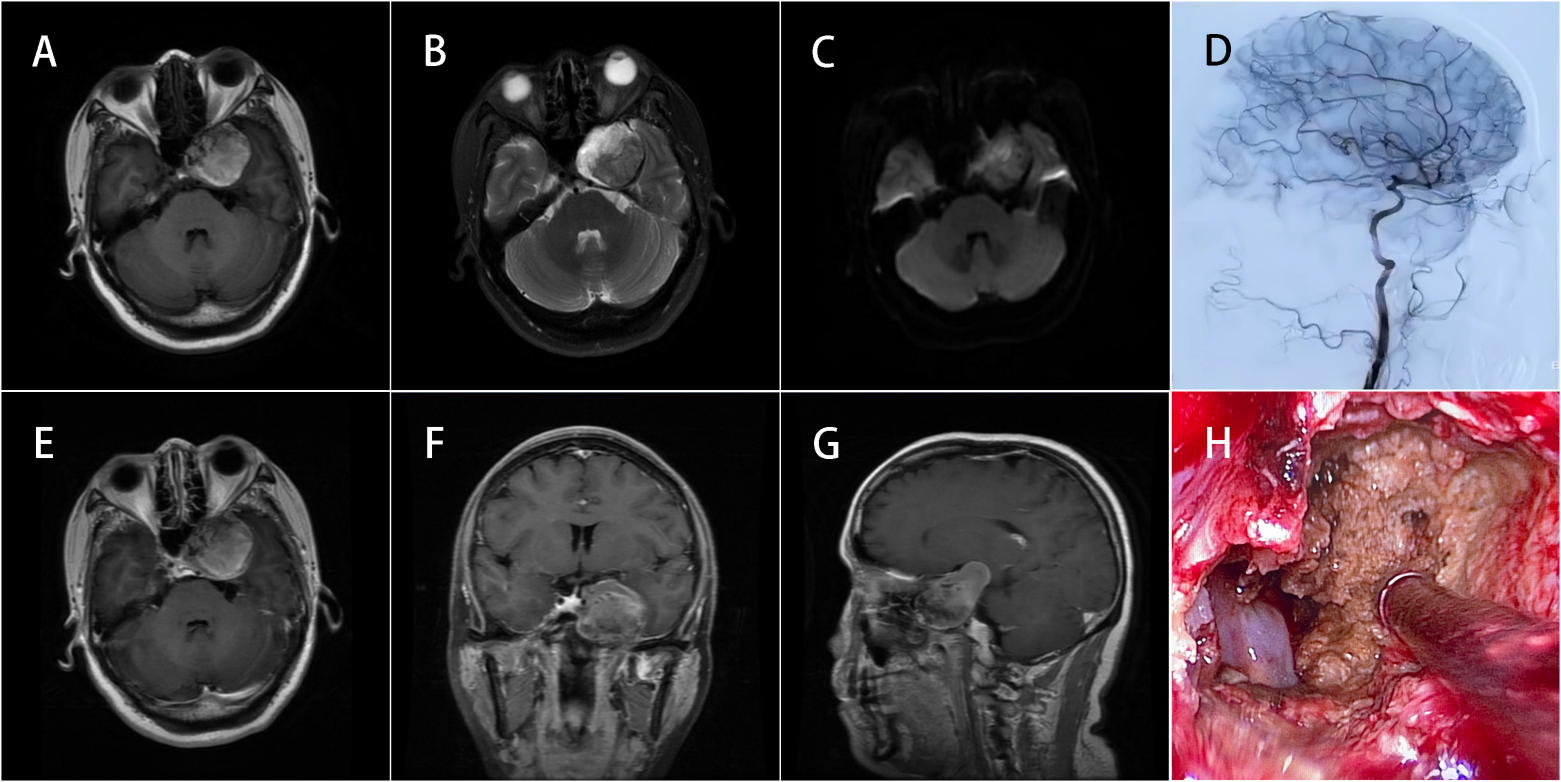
MRI of the brain with and without contrast. (A) The left CS lesion was approximately 34 mm × 30 mm in size with a clear boundary but showed mixed signals that were mostly hyperintense on T1-weighted imaging. (B) Hypointense signals can be seen on T2-weighted imaging. (C) Heterogeneous signals appeared on DWI. (E–G) No obvious gadolinium enhancement was noted. (D) DSA confirmed that the embolization effect on the aneurysm was satisfactory and the shape of other intracranial vessels was smooth. (H) Endoscopic findings revealed that the lesion contents comprised tan mushy substances.
